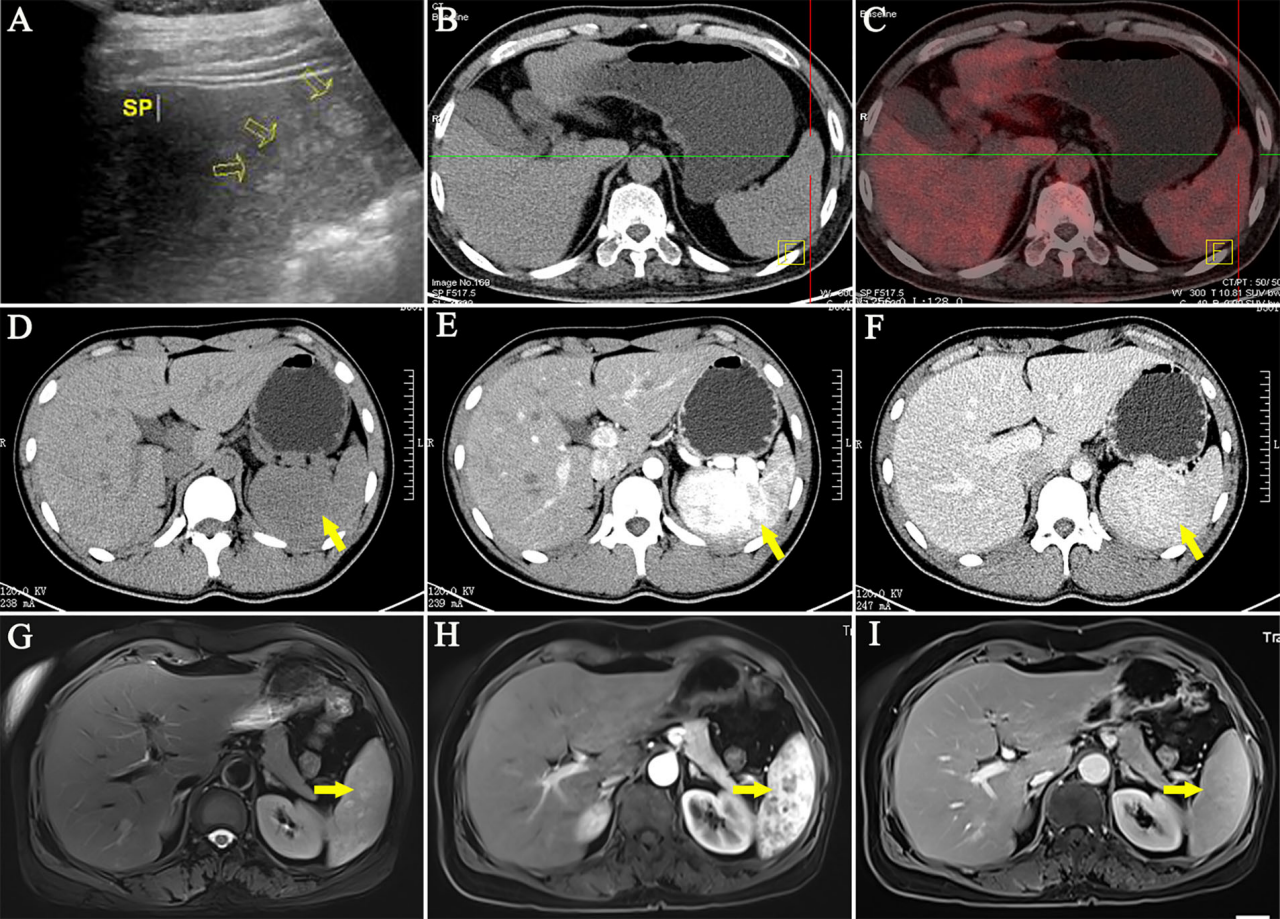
Representative imaging of LCA. (A) Ultrasound imaging often showed hyperechoic lesions. (B, C) The PET/CT showed no abnormal uptake of 18F-FDG. (D) On nonenhanced CT, LCA often manifested as single or multiple hypodense nodules. (E, F) On enhanced CT, LCA characterized by delayed reinforcement. (G) In T2WI, the splenic lesions mostly manifested as a high signal intensity. (H, I) Similar to contrast-enhanced CT, Enhanced MRI showed a delayed enhancement.
